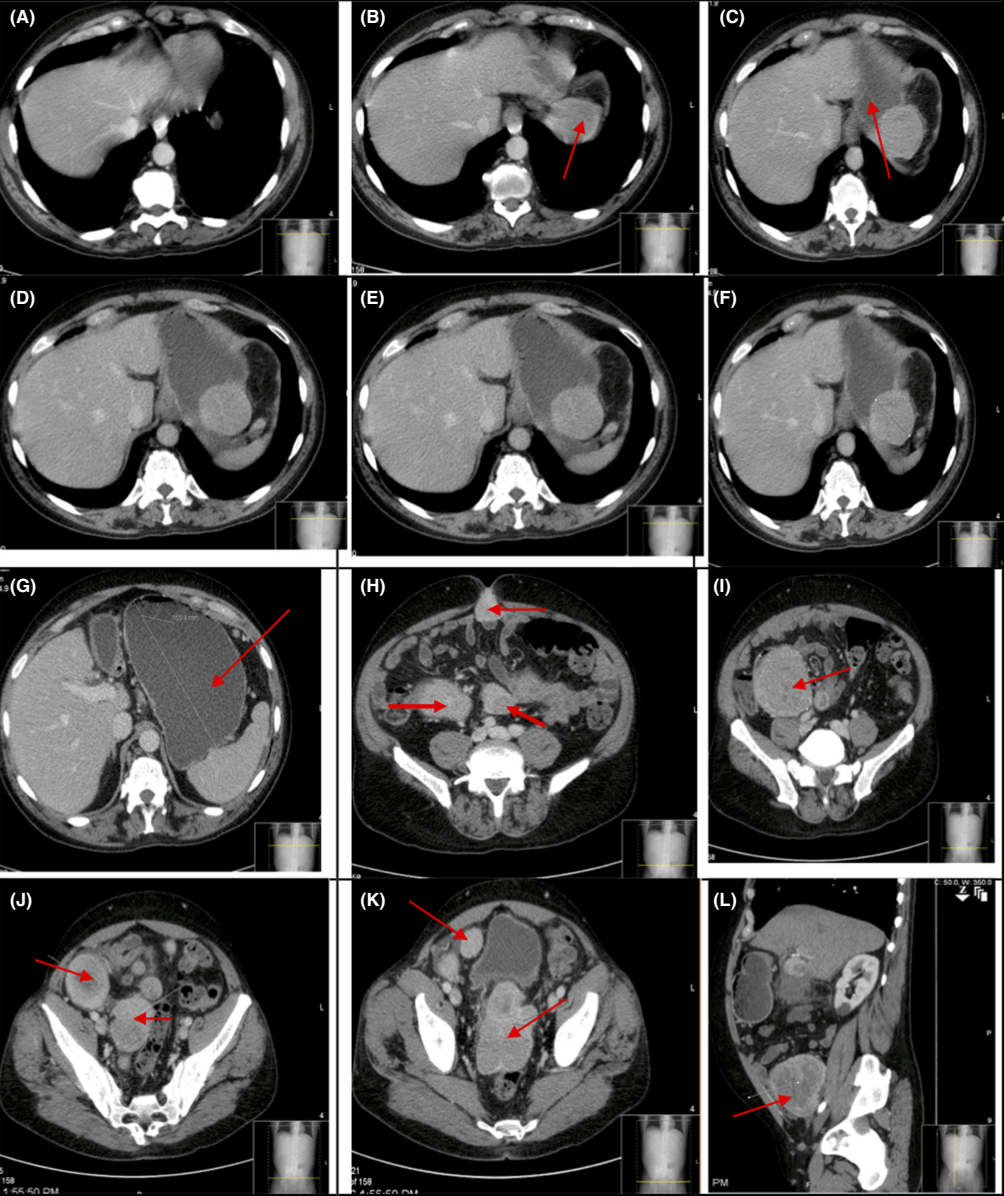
CT abdomen and pelvis after administration of 94 mL of Omnipaque 350 during the arterial phase and portal venous phase. Transverse views are seen A–K. Panels A–G demonstrate different sections of the liver, radiology reviewed as normal in size, and contour with no mass and homogenous attenuation. From Panels B–F, the perigastric mass reviewed as interposed between the left hemidiaphragm and gastric fundus, and measuring 7.6 cm anteroposterior (AP), 5.5 cm transverse, and 5.8 cm craniocaudal. It is marked by an arrow in Panel B. He had extensive fluid in the gastric lumen related to negative oral contrast received prior to examination; this is more prominent in Panel G. Panels H‐K show the multiple abdominal masses (marked by arrows). The largest was reviewed as in the right retroperitoneum, abutting the right psoas muscle, measuring approximately 7.5 cm AP, 9 cm craniocaudal, and 7.5 cm transverse. Panel L show sagittal views of the same CT Scan. Multiple masses can be appreciated, some of which are marked by the arrows.
